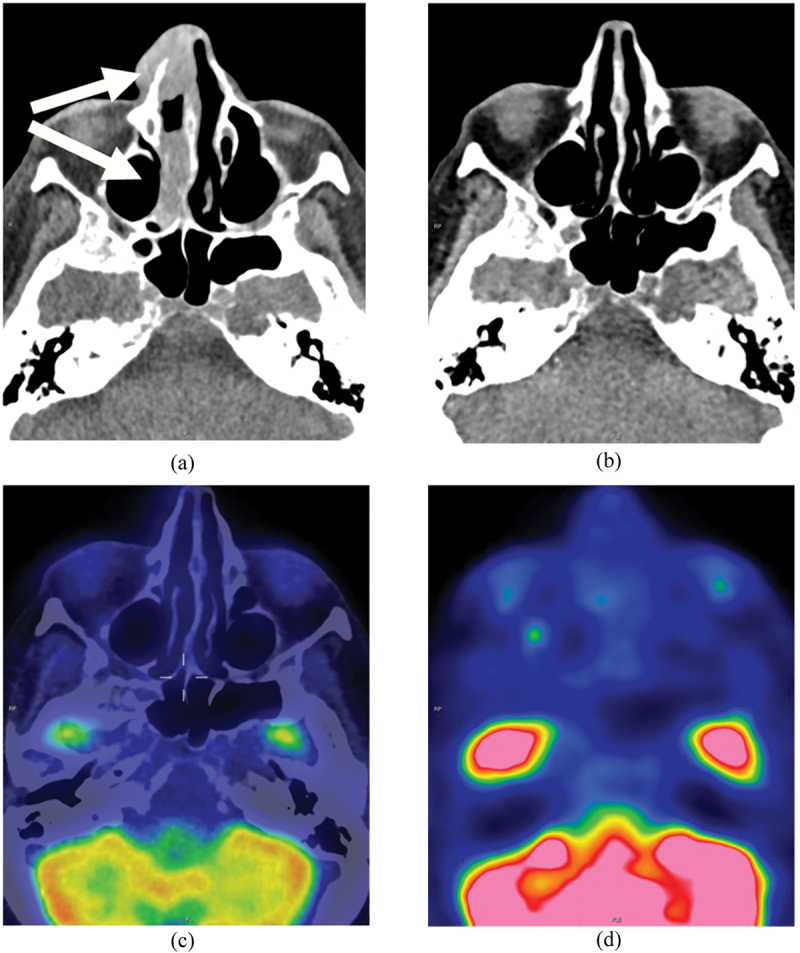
CT sinus at presentation showed soft tissue disease in the nasal cavity and right nasal ala (arrows) (a). CT sinus 3 weeks after methotrexate cessation showed regression of soft tissue disease in right nasal cavity and right nasal ala (b). FDG-PET/CT performed 3 weeks after methotrexate cessation showed disease regression (c, d).
